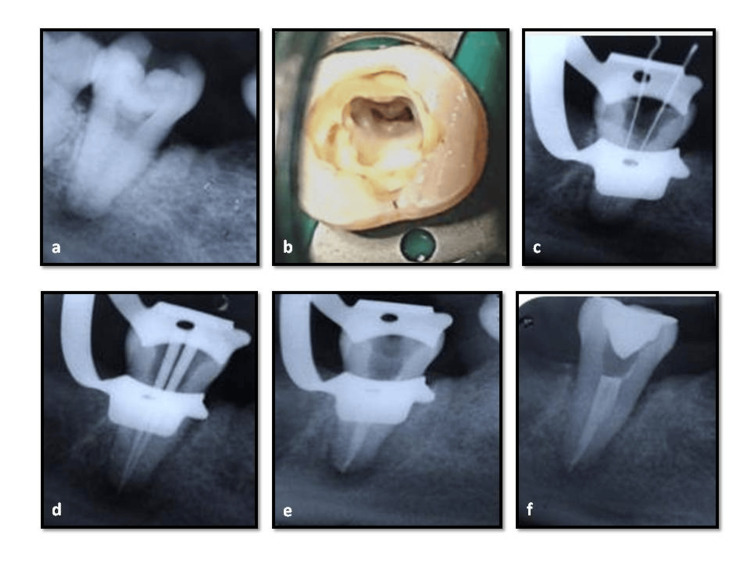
Case report-1 a) Preoperative radiograph shows square-shaped, fused roots; b)access opening, a single, large, c-shaped canal configuration with two orifices interconnected with a isthmus; c)working length radiograph shows narrow isthmus between the mesial and distal canals; d) master cone radiograph; e), f) post-obturation radiographs.
